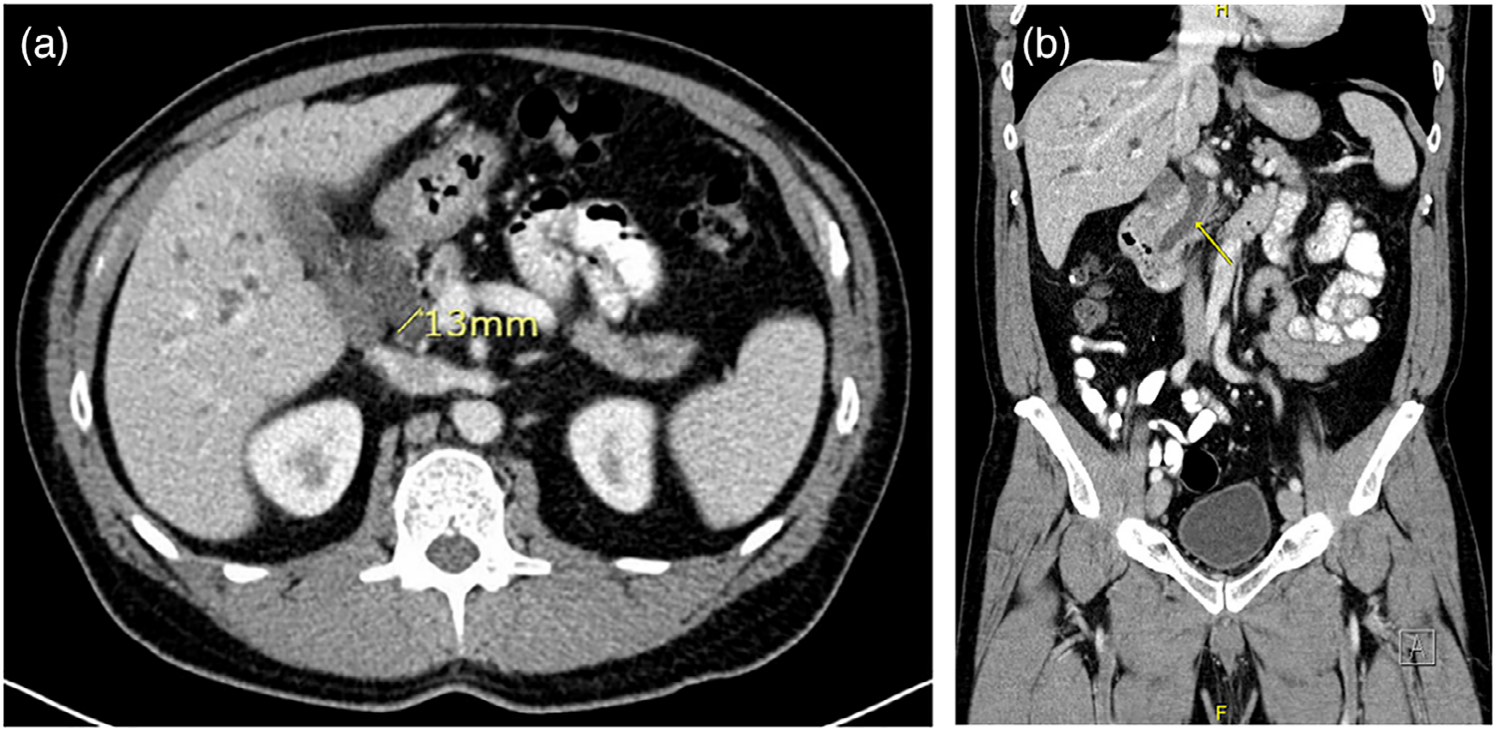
Contrast‐enhanced computed tomography (CT) abdomen with (a) cross‐sectional and (b) coronal views demonstrating significant common bile duct dilatation (>13 mm).
